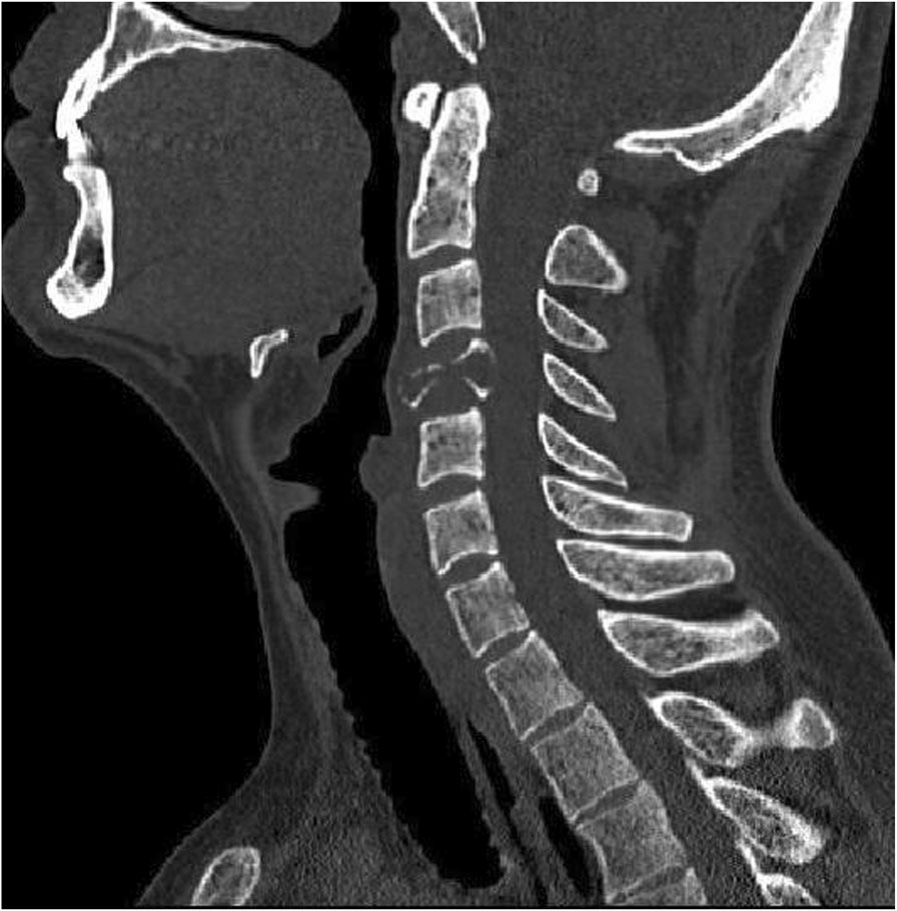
Lytic lesion in C4 vertebral corpus on CT in the sagittal plane
